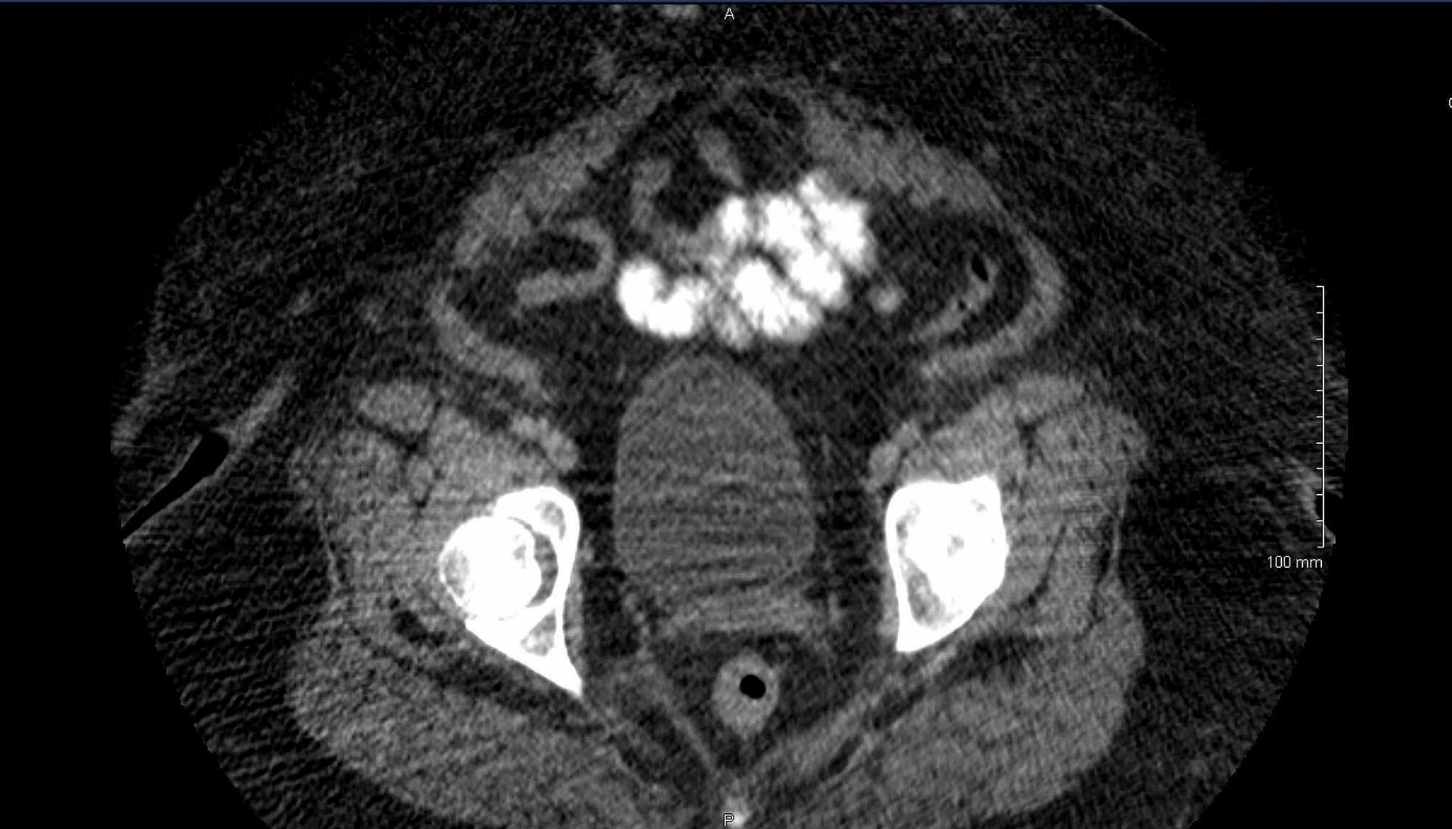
CT pelvis nine months after treatment CT: computed tomography
